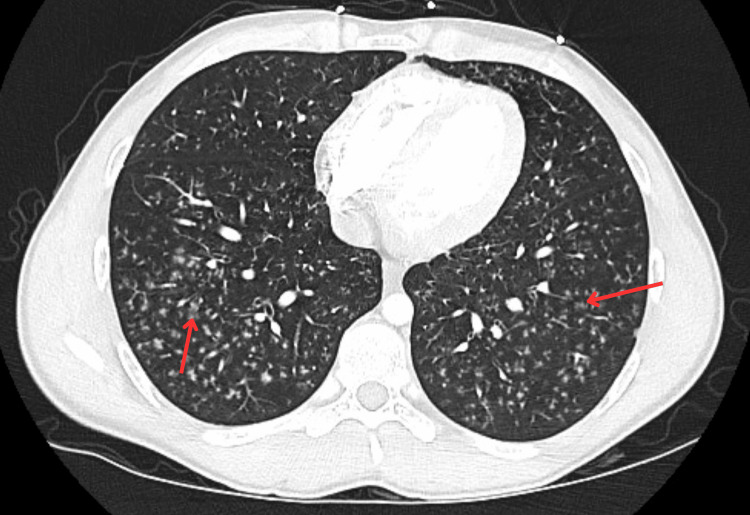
CT chest demonstrating diffuse miliary pulmonary nodules with a "tree-in-bud" pattern (red arrows)
